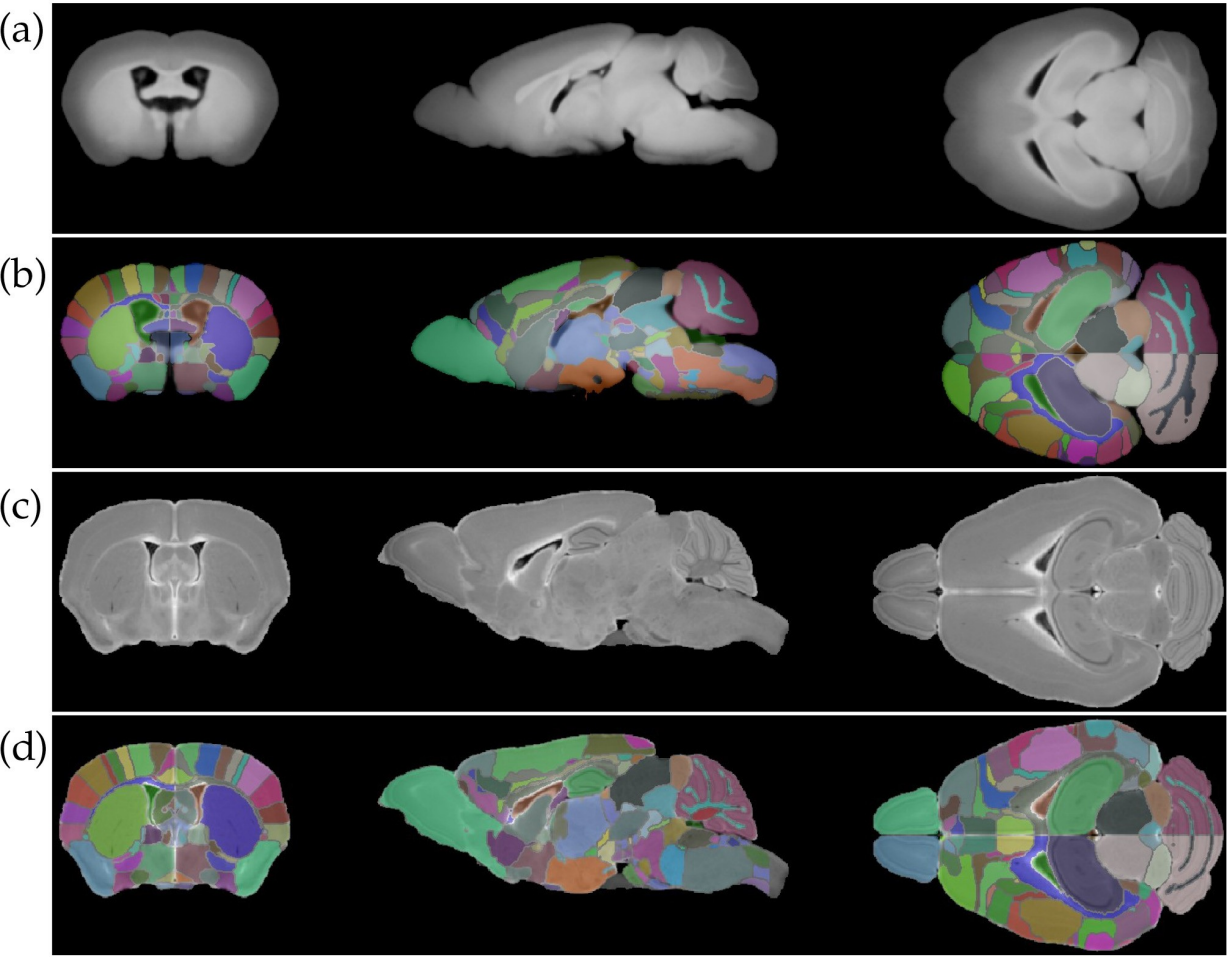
Comparison of atlas brains from PCD CT and DW MRI. (a) PCD 34 keV threshold atlas without labels. (b) PCD 34 keV threshold atlas with labels. (c) DW MRI atlas without labels (d) DW MRI atlas with labels.
